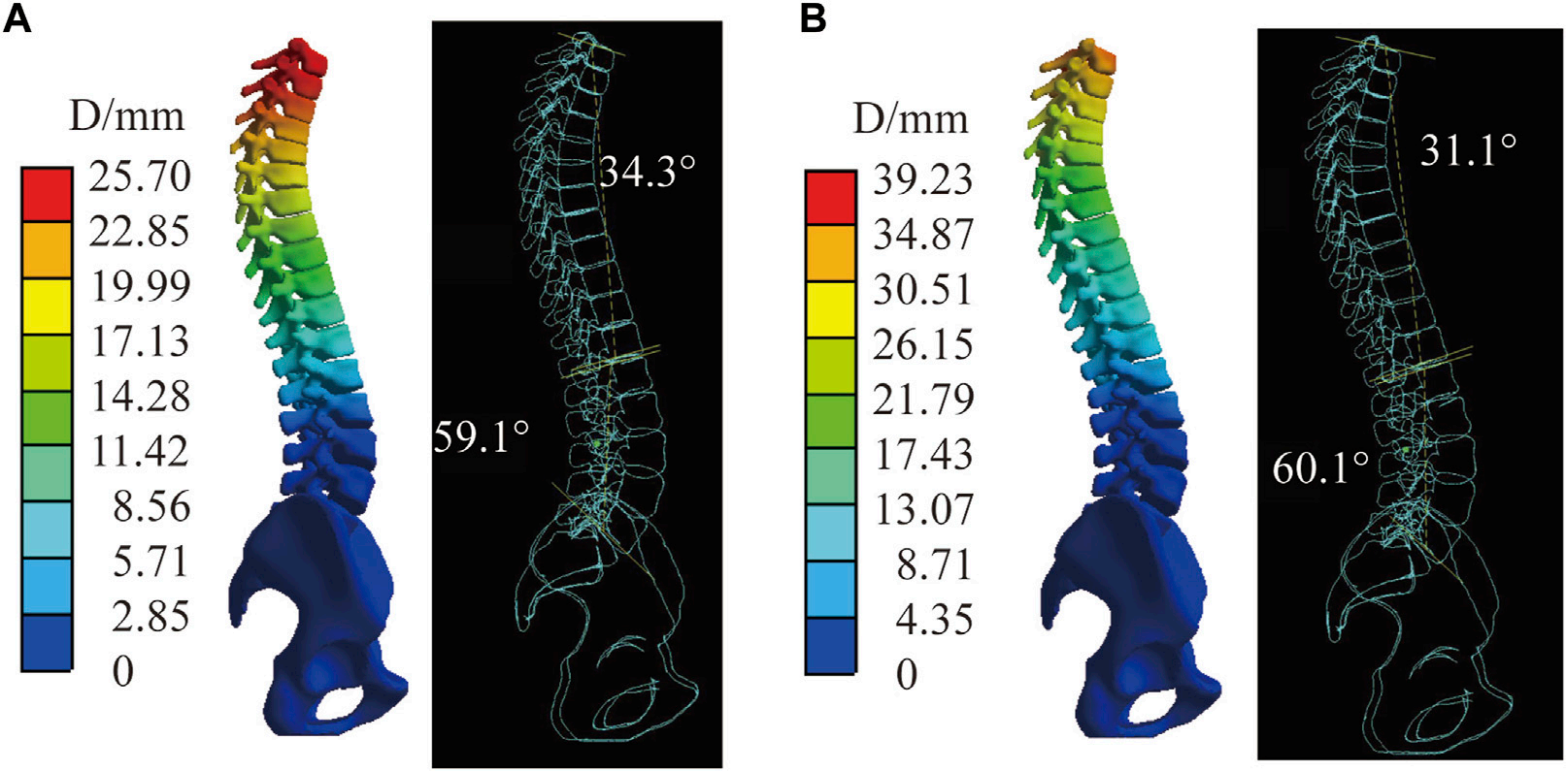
The stiffest spinal in C2 and C4. (A) Displacement distribution and virtual X-ray of sagittal plane in C2. (B) Displacement distribution and virtual X-ray of sagittal plane in C4.
